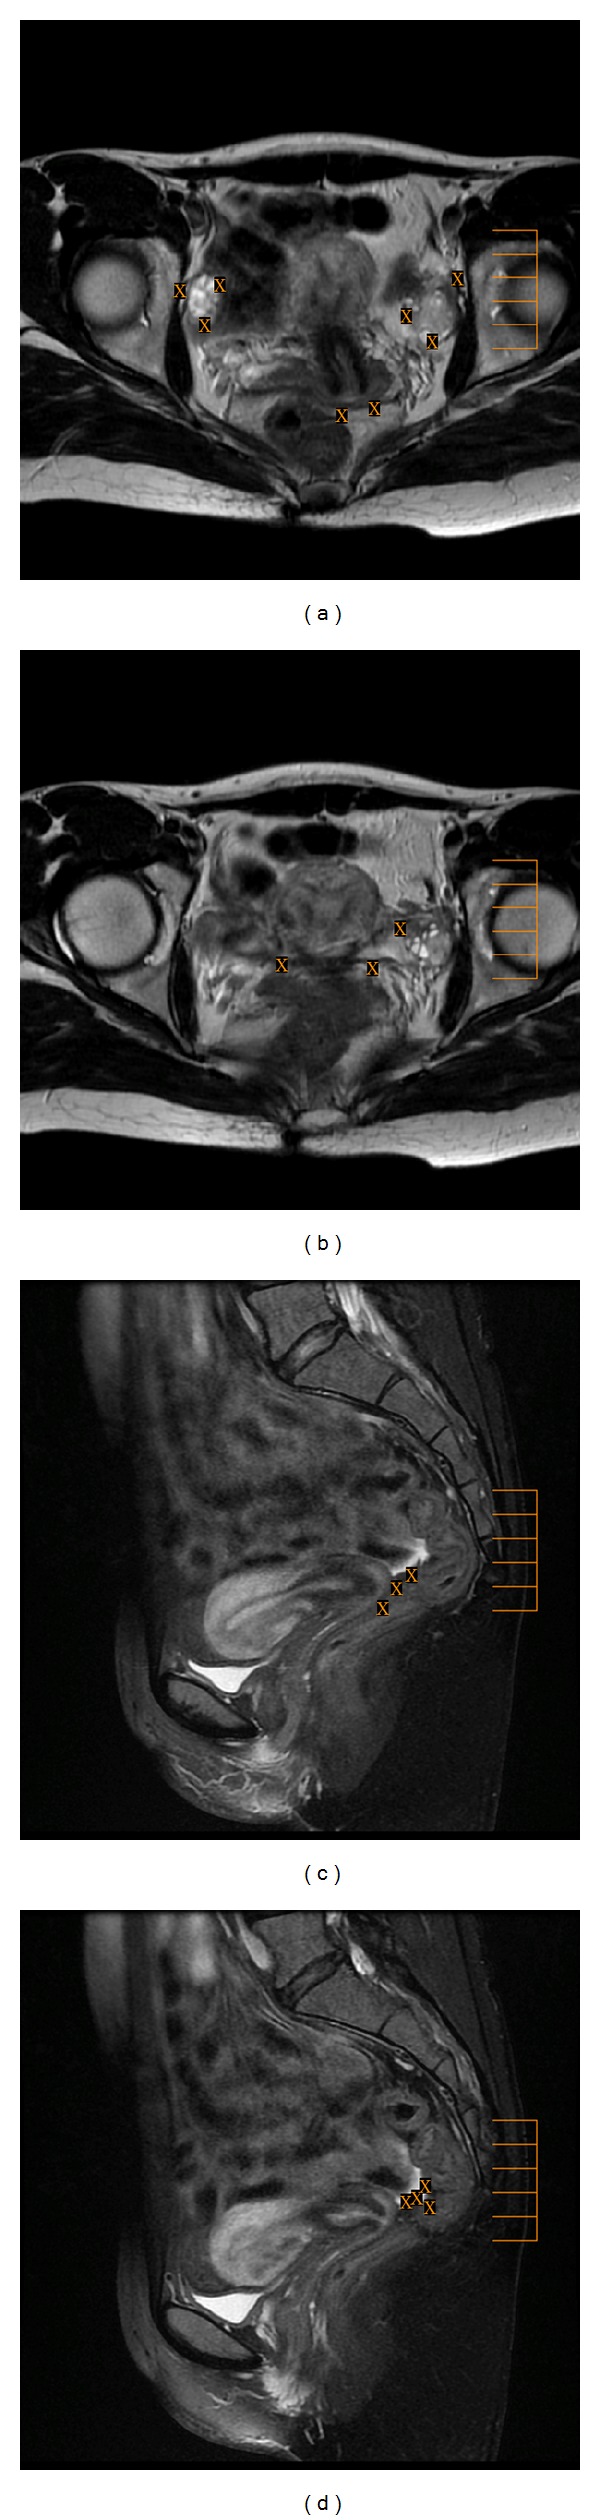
Axial ((a), (b)) T2-weighted fast SE images (repetition time msec/echo time msec = 4500/107) and sagittal ((c), (d)) T1-weighted fast SE images with fat suppression (TR/TE = 660/7.5) show the most frequent sites of involvement with peritoneal endometrial implants (X), such as the surface of the ovaries (a), uterus (a), pouch of Douglas ((a), (d)), uterosacral ligament (b), rectovaginal septum (c), and bowel (d).
